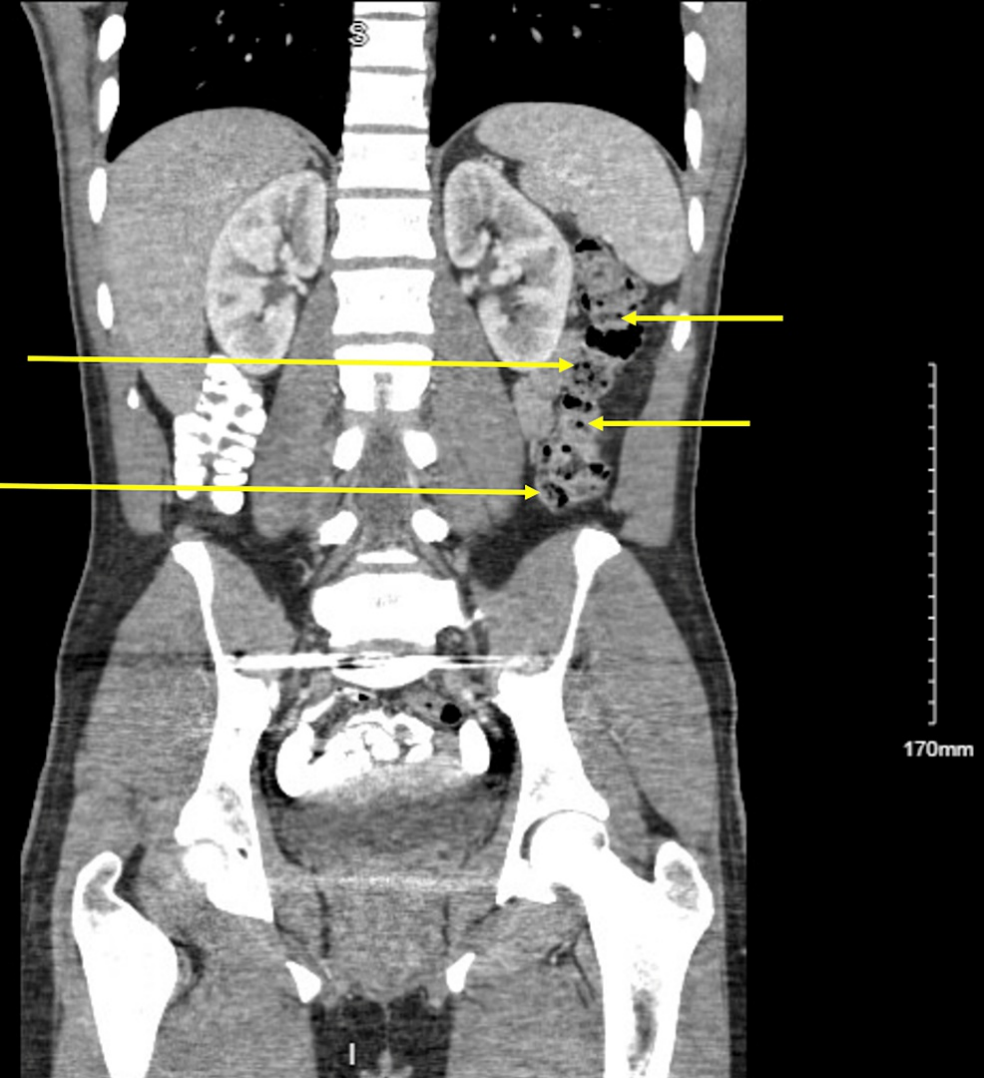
CT abdomen and pelvis demonstrating several filling defects throughout the ileum (arrows).
